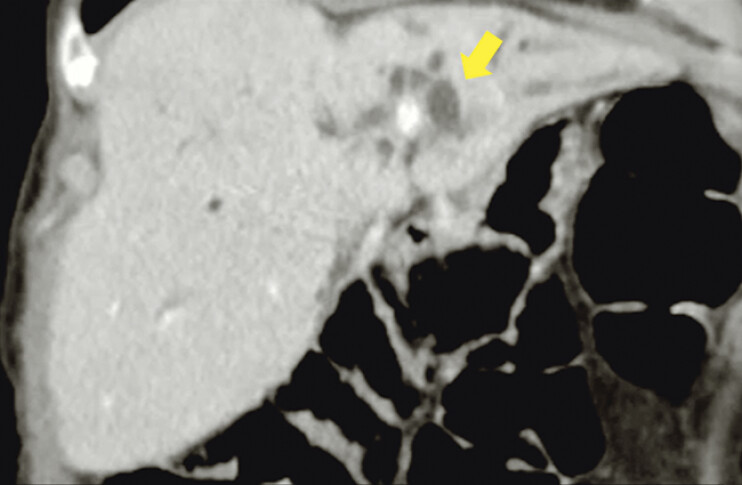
Follow-up CT scan showing resolution of the cystic lesion with persistent dilatation (arrow) of the bile ducts.
